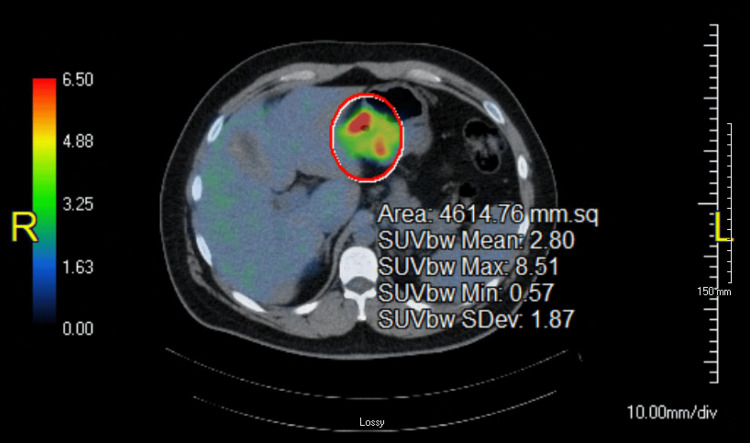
PET scan showing an annular mass in the gastric antrum with an area of approximately 75 mm, with maximum SUV of 8.5 PET: positron emission tomography; SUV: standardized uptake value
